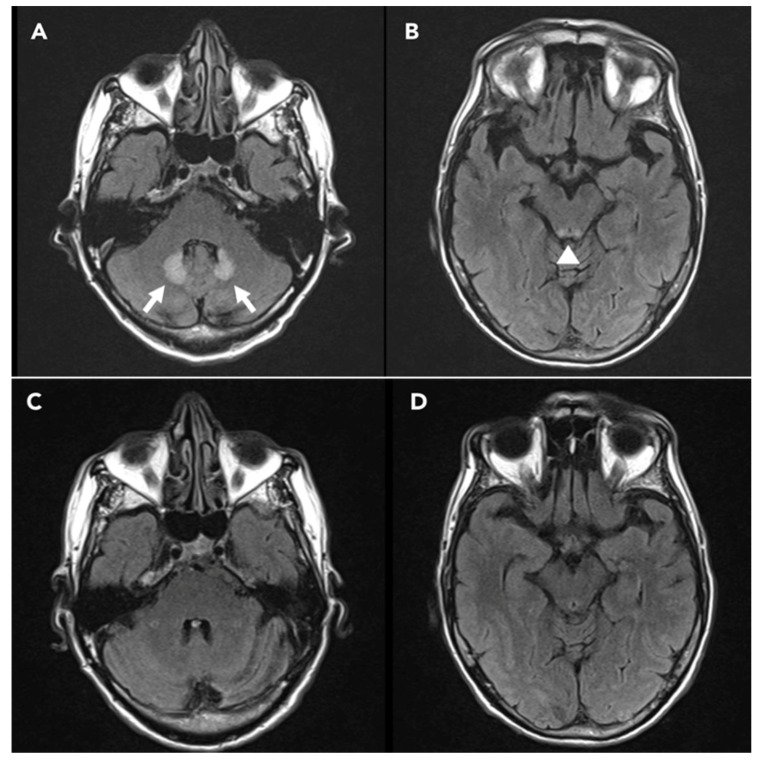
A 1.5 Tesla brain MRI. Fluid-attenuated inversion recovery (FLAIR) sequences showing hyperintensity in (A) bilateral dentate nuclei of the cerebellum (arrows) and (B) dorsal midbrain (arrowhead). A follow-up MRI performed one month after metronidazole discontinuation did not show any abnormalities (C,D).
